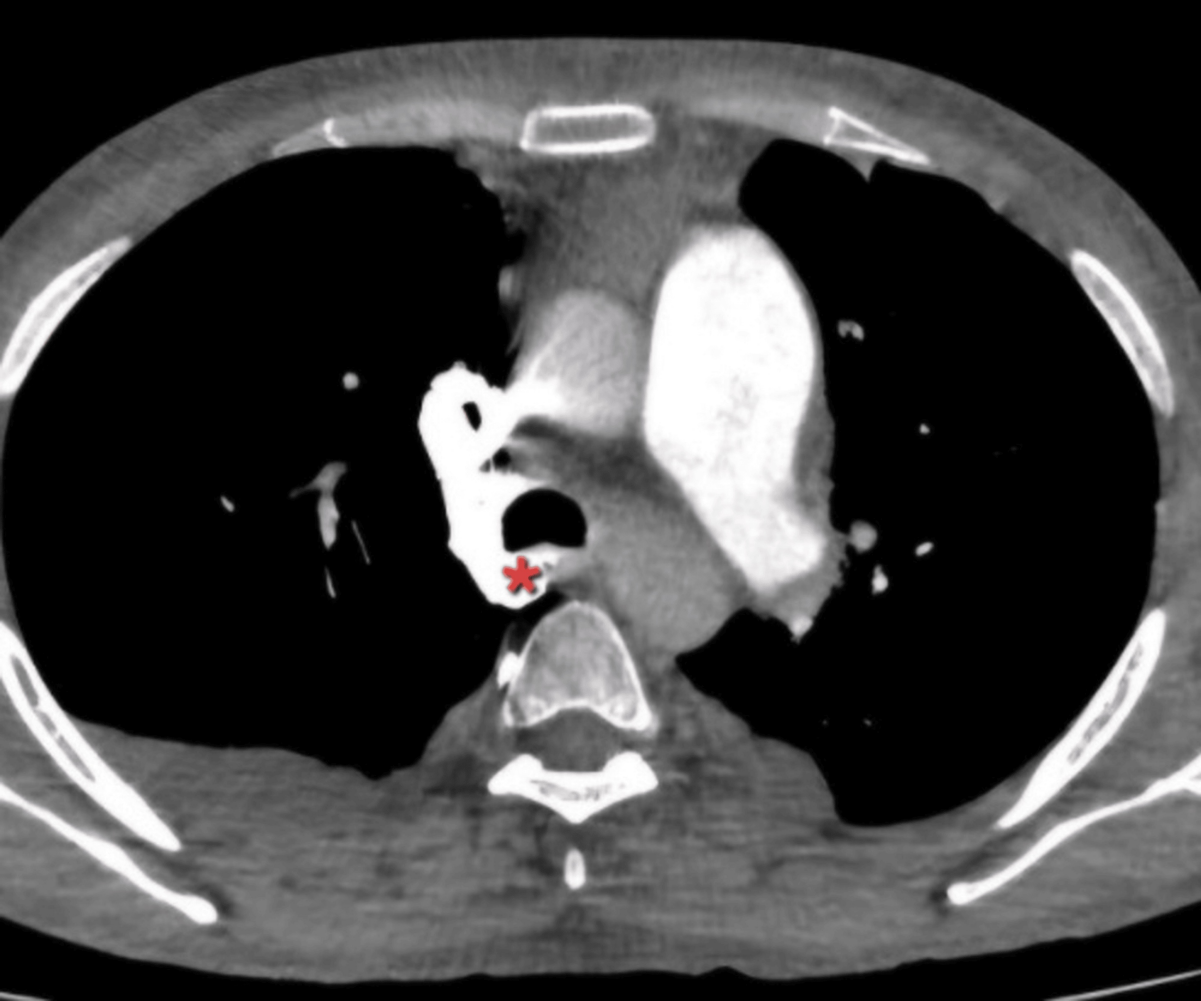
CT chest demonstrating dilated azygos vein measuring 12.1mm (red asterisk)
